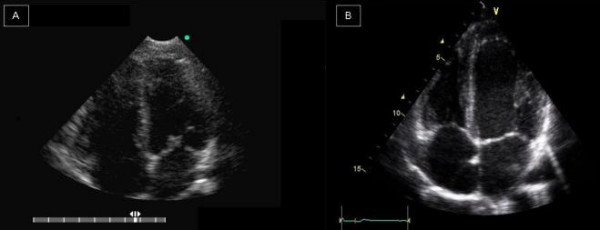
A normal patient. On the left, it’s shown an apical four-chambers view obtained with HHD (A). On the right (B), the same patient evaluated with SED.
